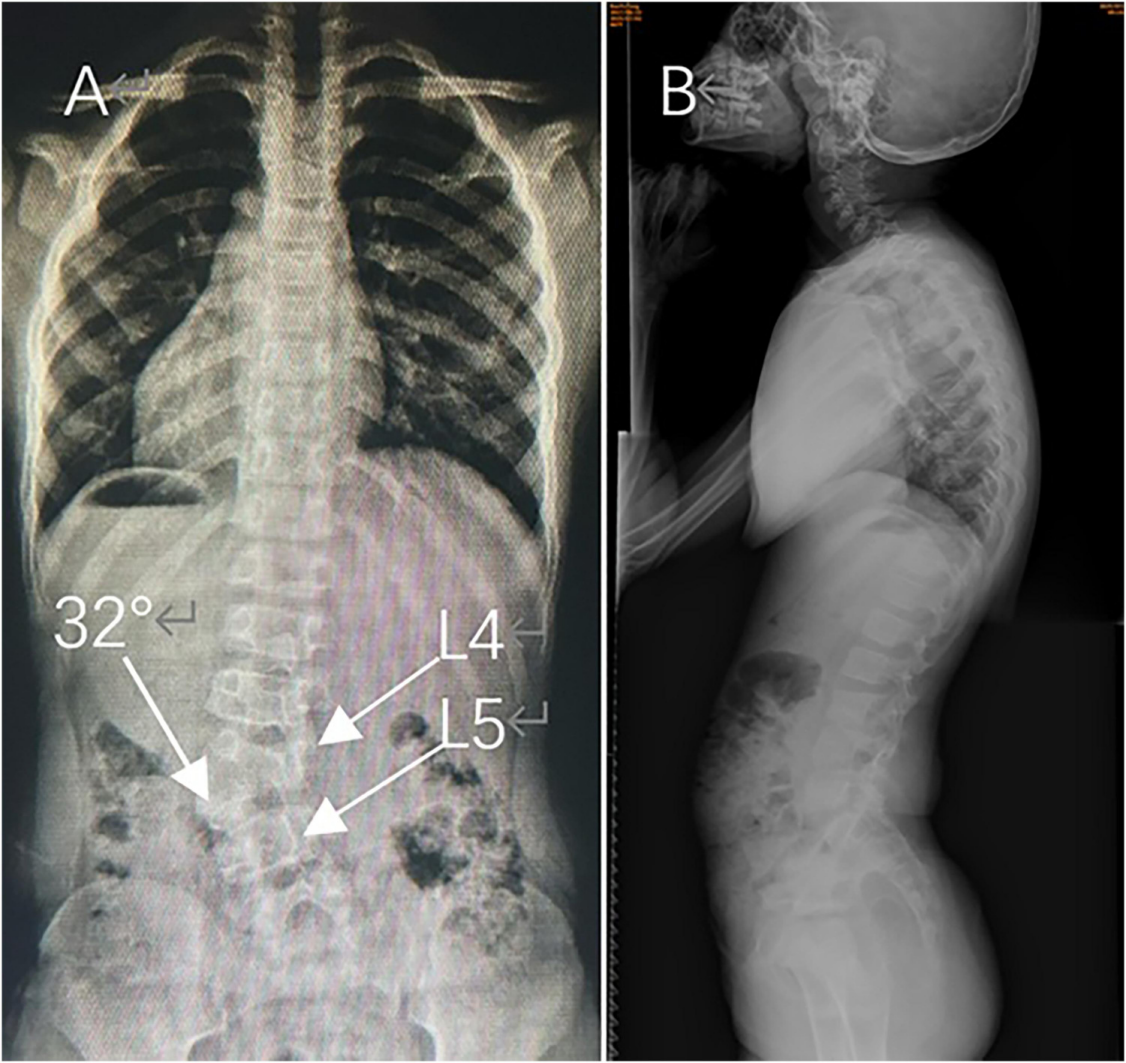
Preoperative full-spine standing radiographs. (A) Anteroposterior (AP) view demonstrating CS with a left L4/L5 semi-segmented HV (white arrow), a segmental Cobb angle of 32°, and compensatory sacral obliquity. (Levels L4 and L5 are labeled.) (B) Lateral view showing preserved physiological lumbar lordosis.
